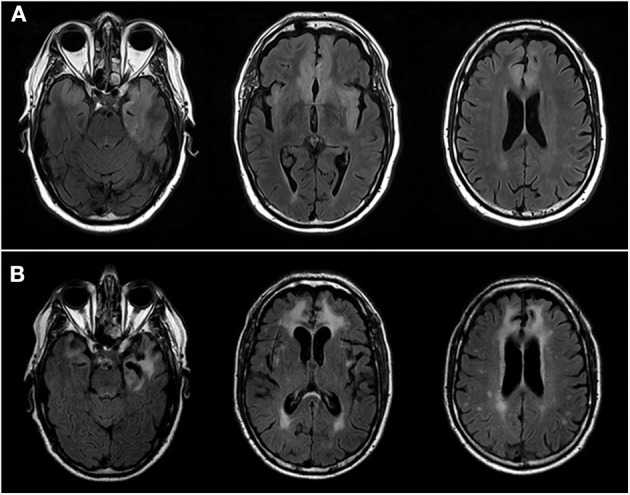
3T Brain MRIs. (A) Patient N°1 MRI at the acute phase of the herpetic meningoencephalitis. Axial images showing hypersignal of the anterior, intern, left, temporal lobe including uncus and hippocampus, left insula and bilateral, ventromedial, orbito-frontal and cingulate anterior lobes. (B) Patient N°1 MRI at the time of AIE. Axial FLAIR images showing sequels of herpetic meningoencephalitis, including extension of previously describe lesions and necrosis of the medial, anterior, left, temporal lobe. Of note, increase of vasculoderenerative subcortical, bilateral lesions.
